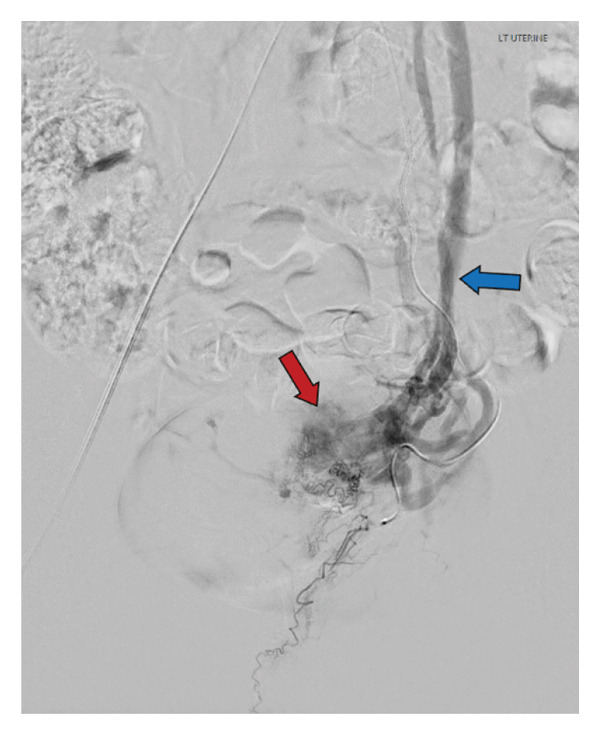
Left uterine artery angiogram confirming CTA findings, showing multiple small serpentine branches (red arrow) arising from the uterine artery with early venous return (blue arrow).
